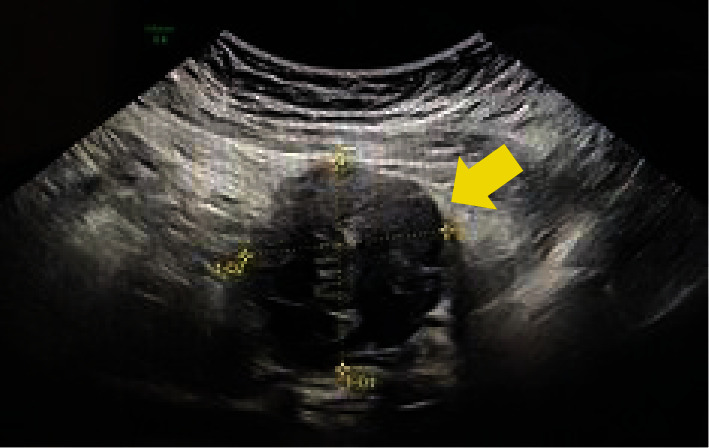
Image of the subserosal fibroid measuring 3.8 × 3.2 × 4.0 cm on the patient's initial ultrasound performed at 10-week gestational age.
